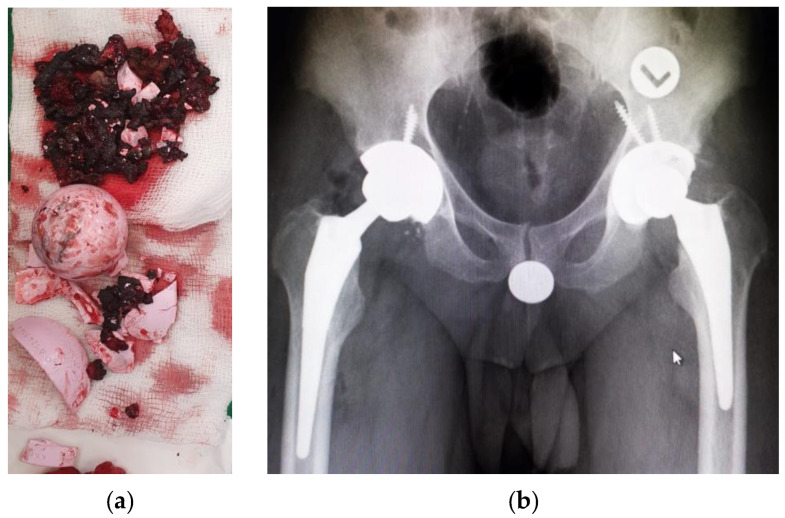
(a) Fractured ceramic liner and extracted debris during surgery; (b) X-ray showing right implant after the second THA.
